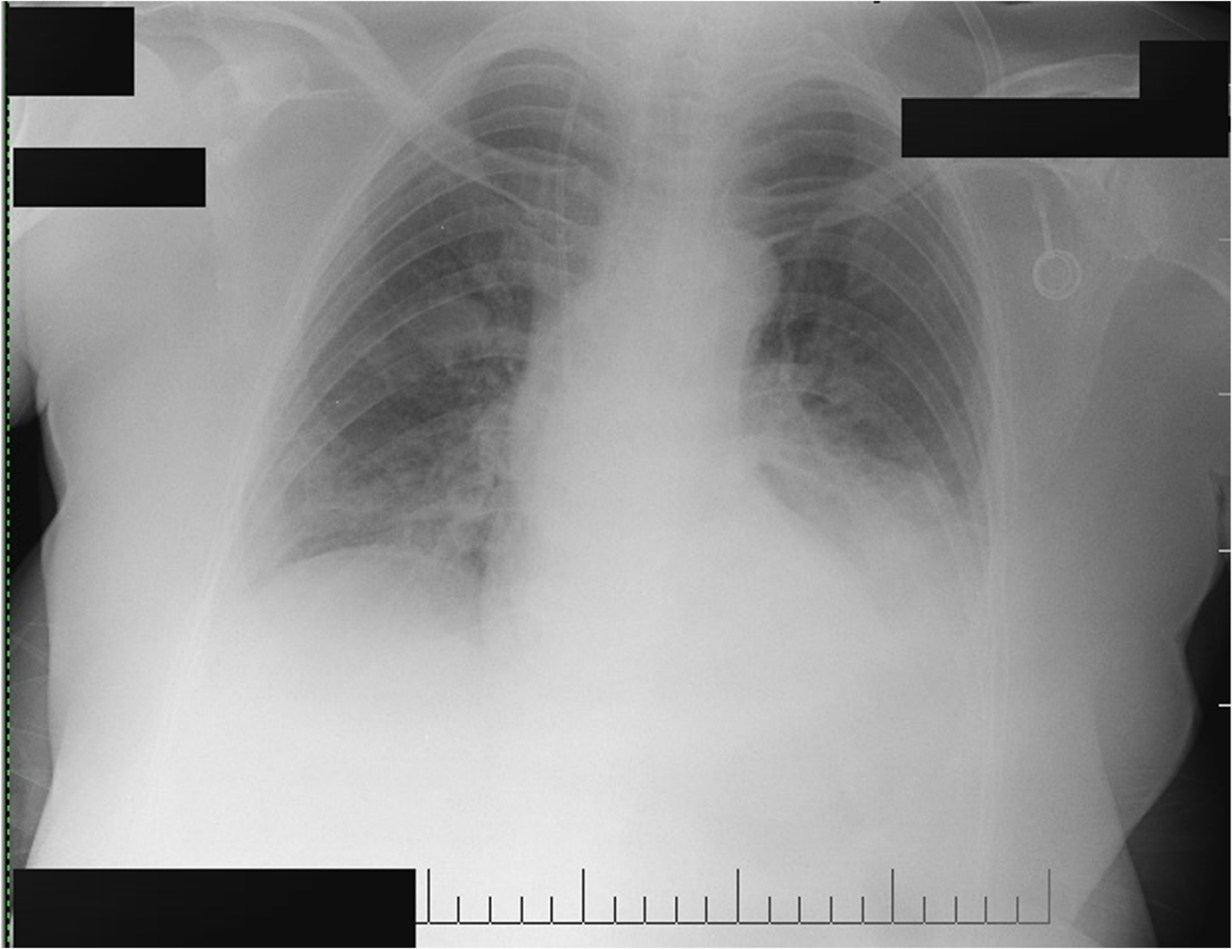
Chest X-rays after extubation (day 20 of ICU). The regression of the bilateral infiltrates and mild bilateral pleural effusion can be noticed
